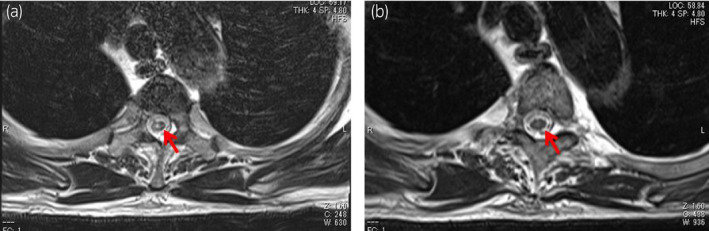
Spinal magnetic resonance imaging showed T2WI hyperintensities below Th2 levels (arrow) disappeared after two cycles of steroid‐pulse therapy. (a) Onset of myeloradiculoneuropathy. (b) After two cycles of steroid‐pulse therapy.
